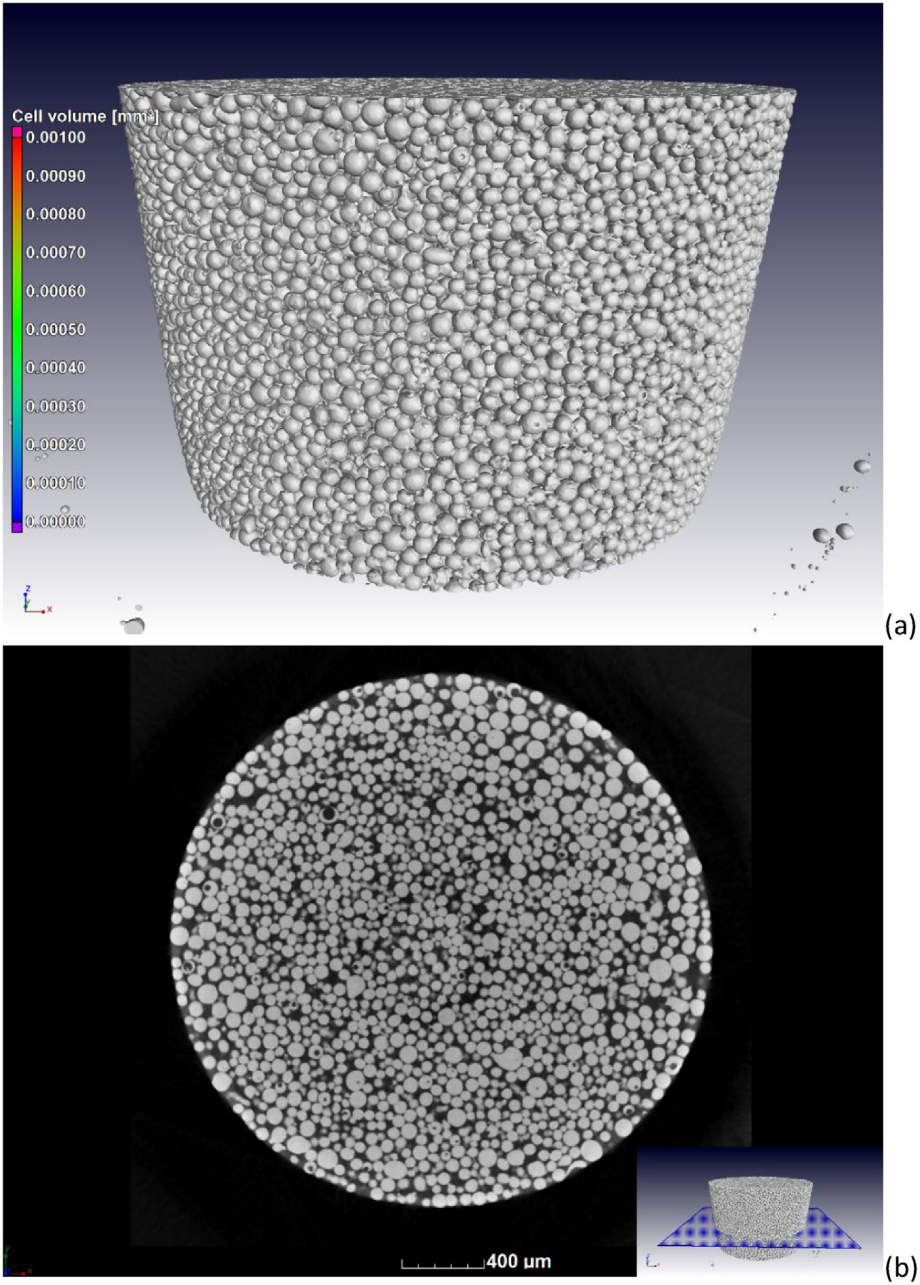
CT scan results showing (a) 3D surface view and (b) CT slice image clearly indicating particles with pore spaces (black circles). Visualizations performed with VGStudio MAX 3.1.
